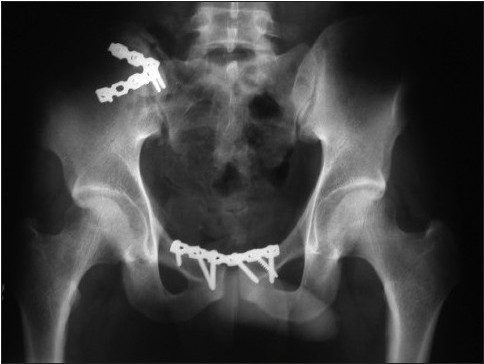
Three years postoperative radiograph of the patient showing well-maintained fixation. The patient was ambulating well without any symptoms
